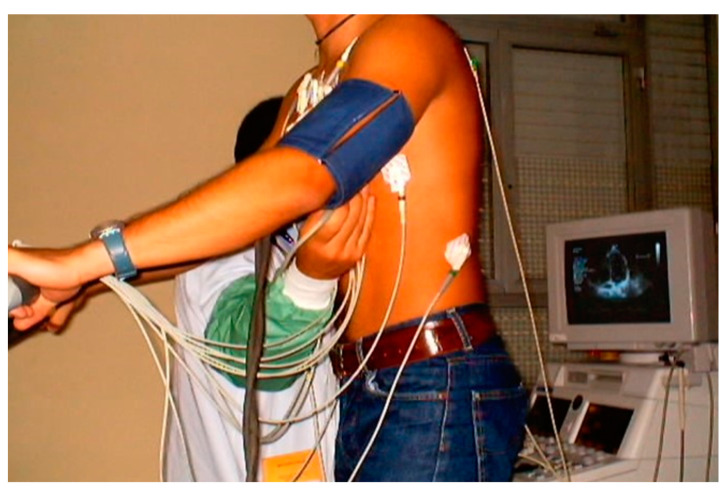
Image acquisition in the orthostatic position during exercise stress echocardiography using a treadmill for a 17-year-old boy with chest pain related to exercise.
